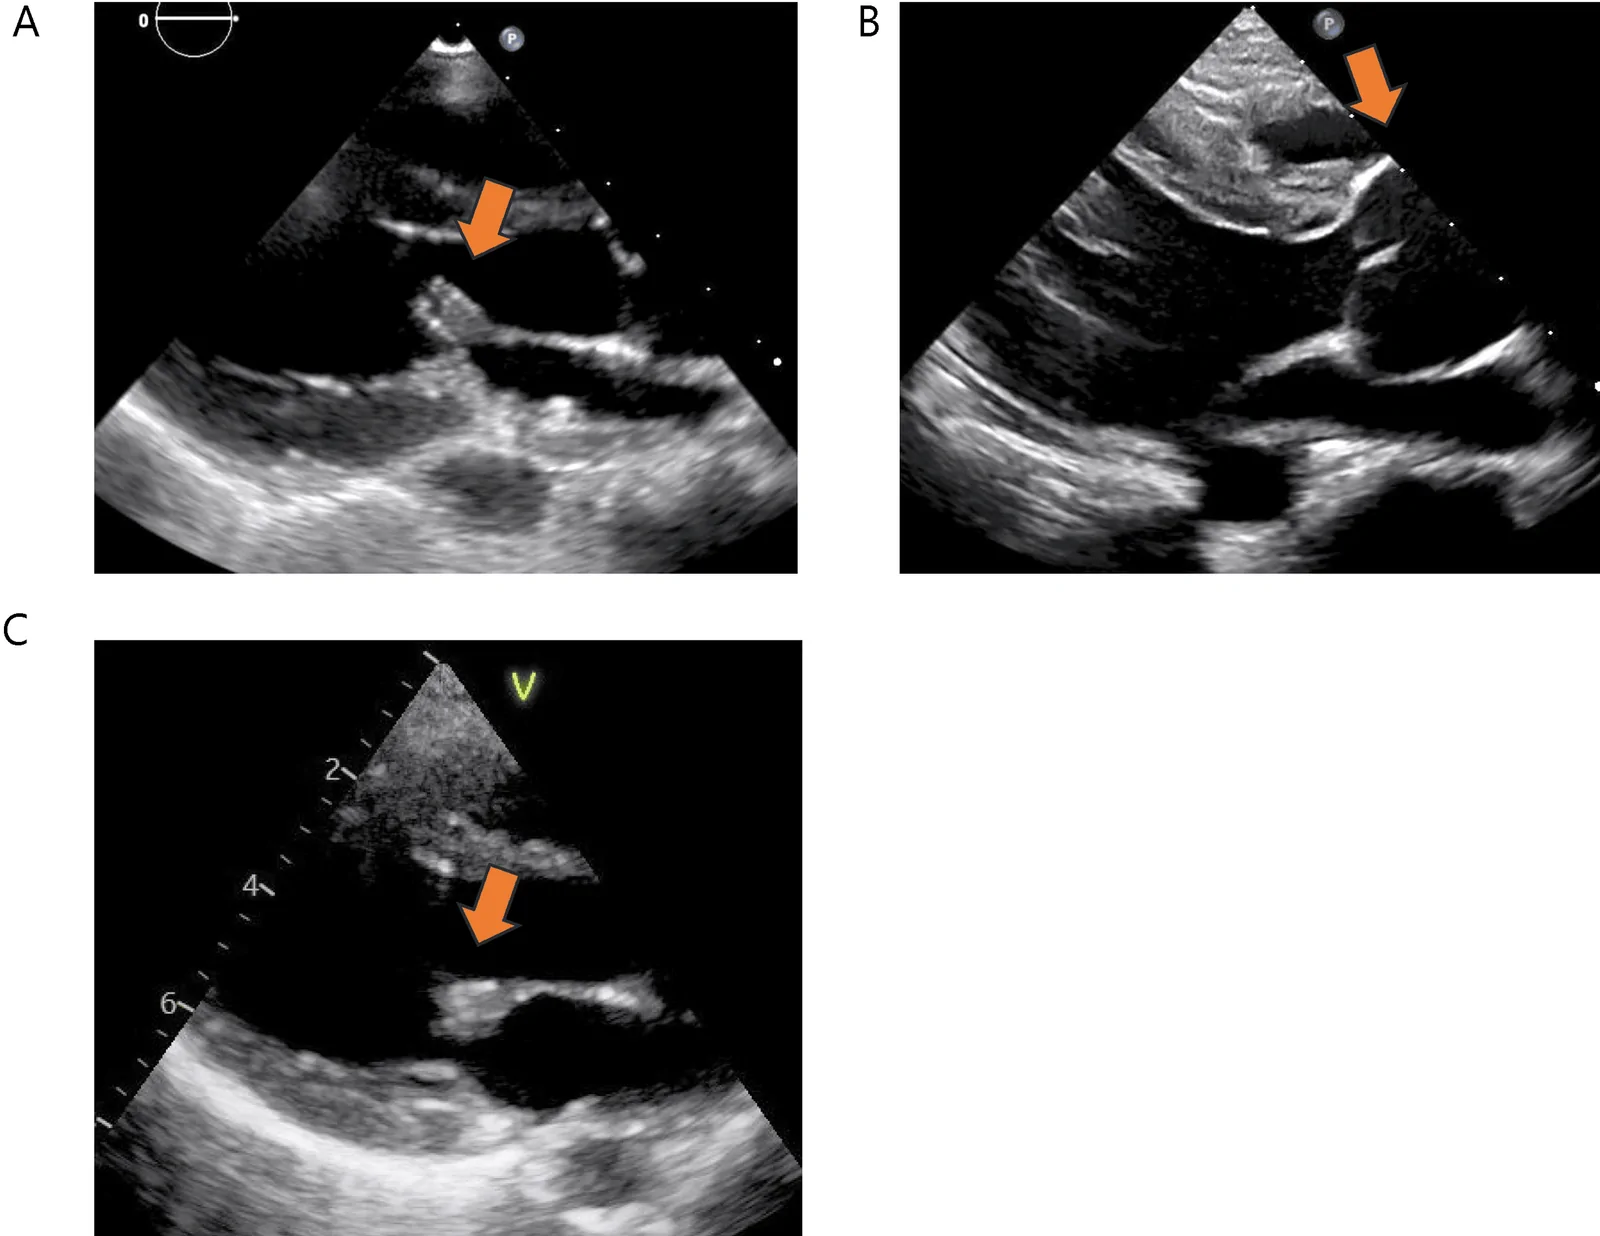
Echocardiography (A) Left parasternal long-axis view on admission shows an infective endocarditis (IE) vegetation-like structure on the anterior leaflet of the mitral valve. (B) Left parasternal long-axis view on admission also shows enlargement of the aortic sinus of Valsalva. (C) IE vegetation-like structure was recorded in a previous echocardiographic evaluation before the onset of this infection.
